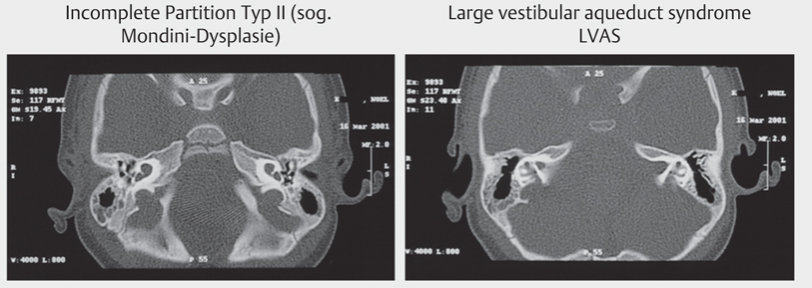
Cone beam tomography of the temporal bone
